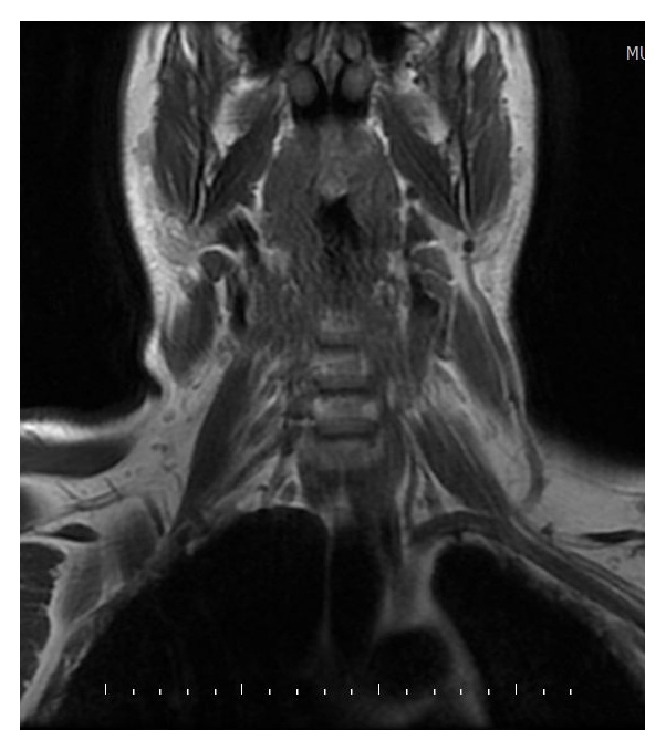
MRI was normal at the postoperative period.
